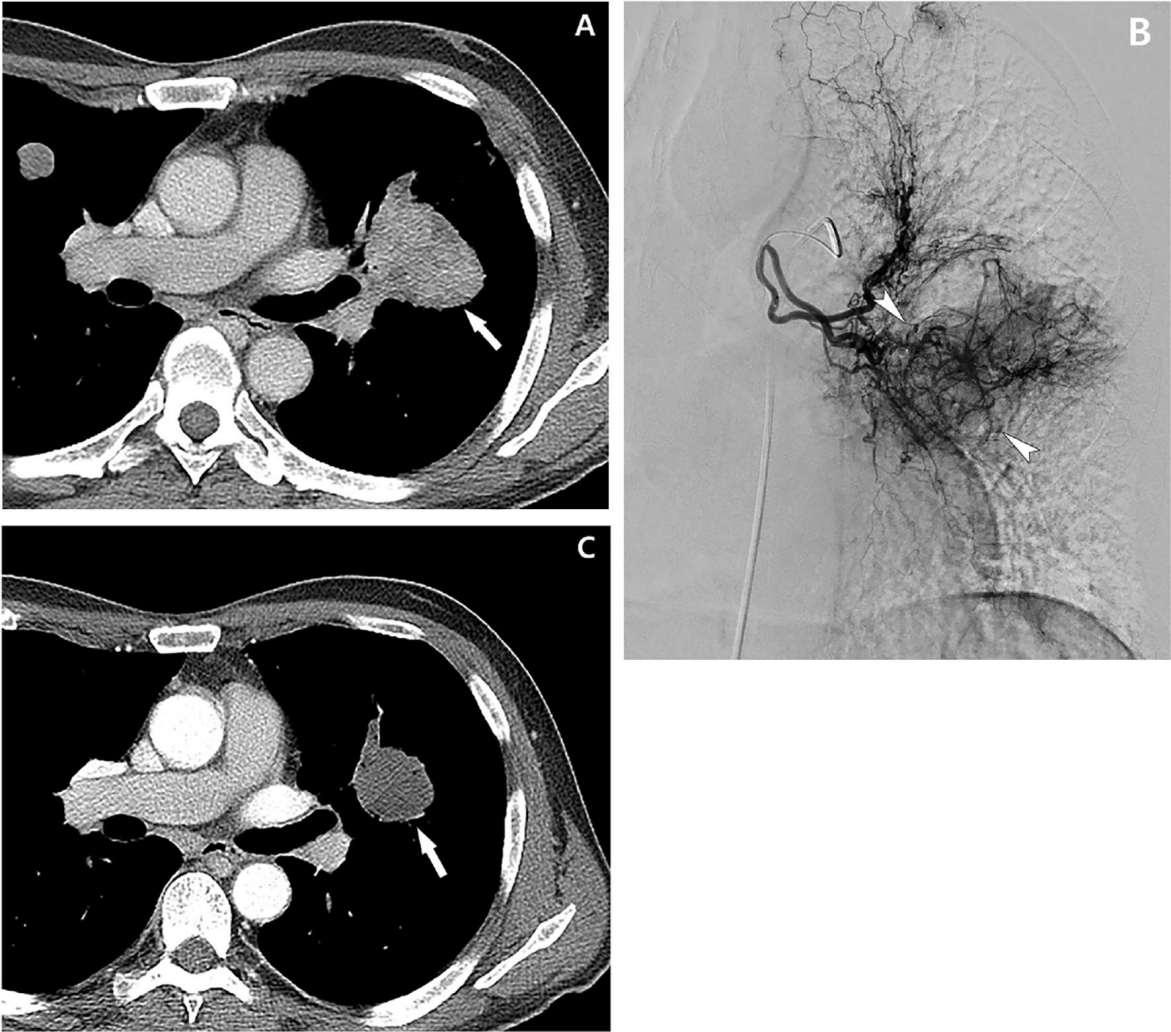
A 61-year-old man with pulmonary metastasis from HCC who presented with a moderate amount of hemoptysis. (A) Contrast-enhanced axial CT image before embolization shows a well-enhancing mass (largest metastatic tumor, 5 cm in diameter, white arrow) in the left lower lobe of the lung. (B) Selective left bronchial arteriography shows a tortuous hypertrophic left bronchial artery and large hypervascular tumor blush (white arrowheads) corresponding to the largest metastatic tumor on CT. Embolization of the left bronchial artery was successfully performed using PVA, and this patient showed complete resolution of hemoptysis after bronchial artery embolization. (C) Contrast-enhanced axial CT image 1 month after embolization of the bronchial artery shows that the largest metastatic mass has decreased in size (white arrow, decreased from 5 to 3 cm in diameter) and seems to be devascularized after bronchial artery embolization.
